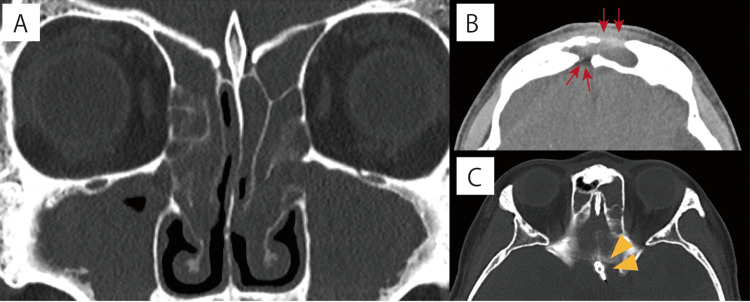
Preoperative CT scan A and B: There was a notable thickening of the subcutaneous tissue in the forehead; partial loss of the frontal bone and partial bony fusion at the posterior wall of the frontal sinus were observed (red arrows). C: Presence of a surgical clip (yellow triangles). CT: computed tomography
